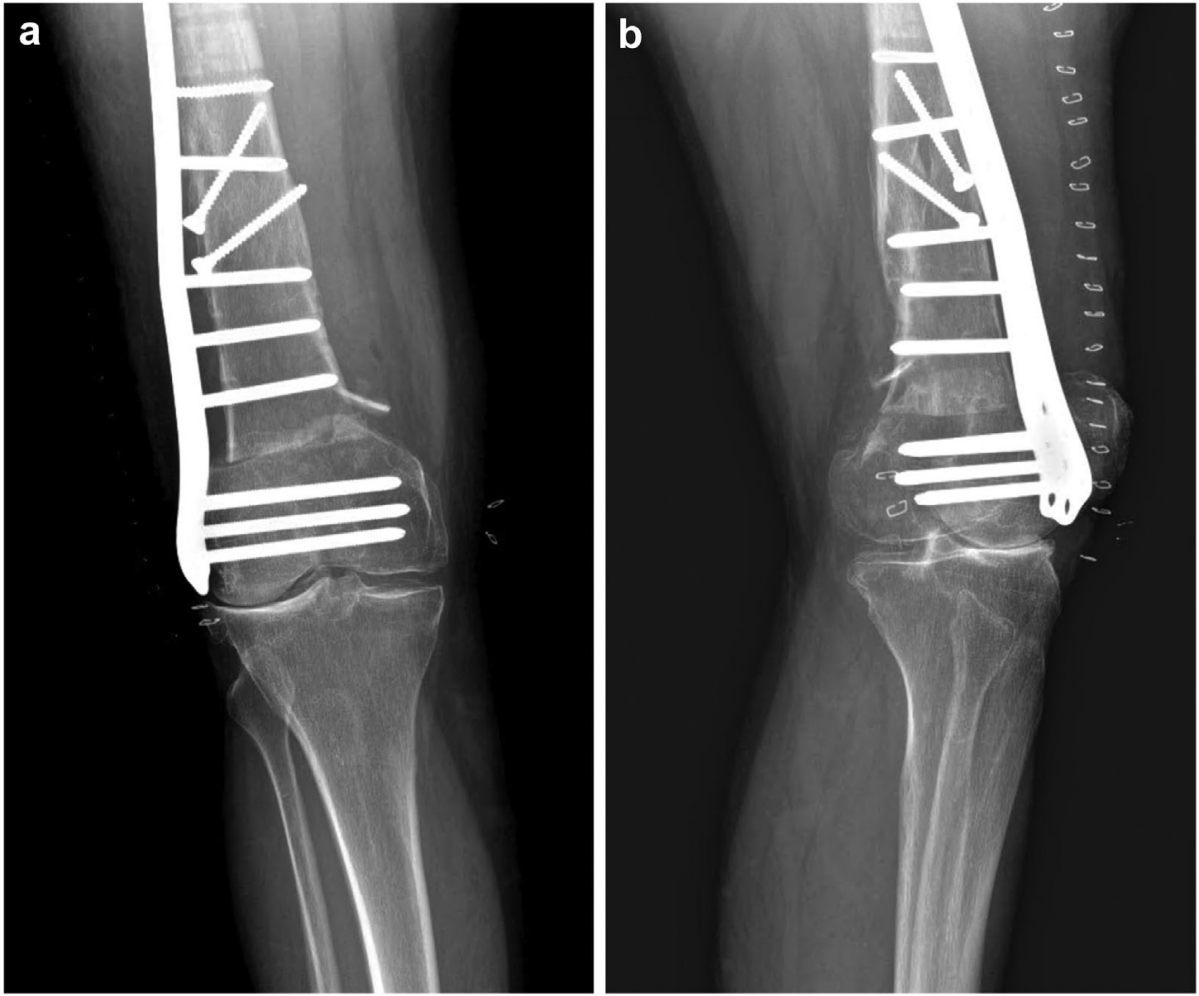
Condition of the femur after the first LCP-DF extraction with a subsequent distal varisation osteotomy, followed by another LCP-DF fixation. A medial cortex osteoporotic compressive fracture of the proximal fragment is visible in the proximity of the osteotomy. (a) Anteroposterior radiograph; (b) TKA detail, lateral radiograph.
